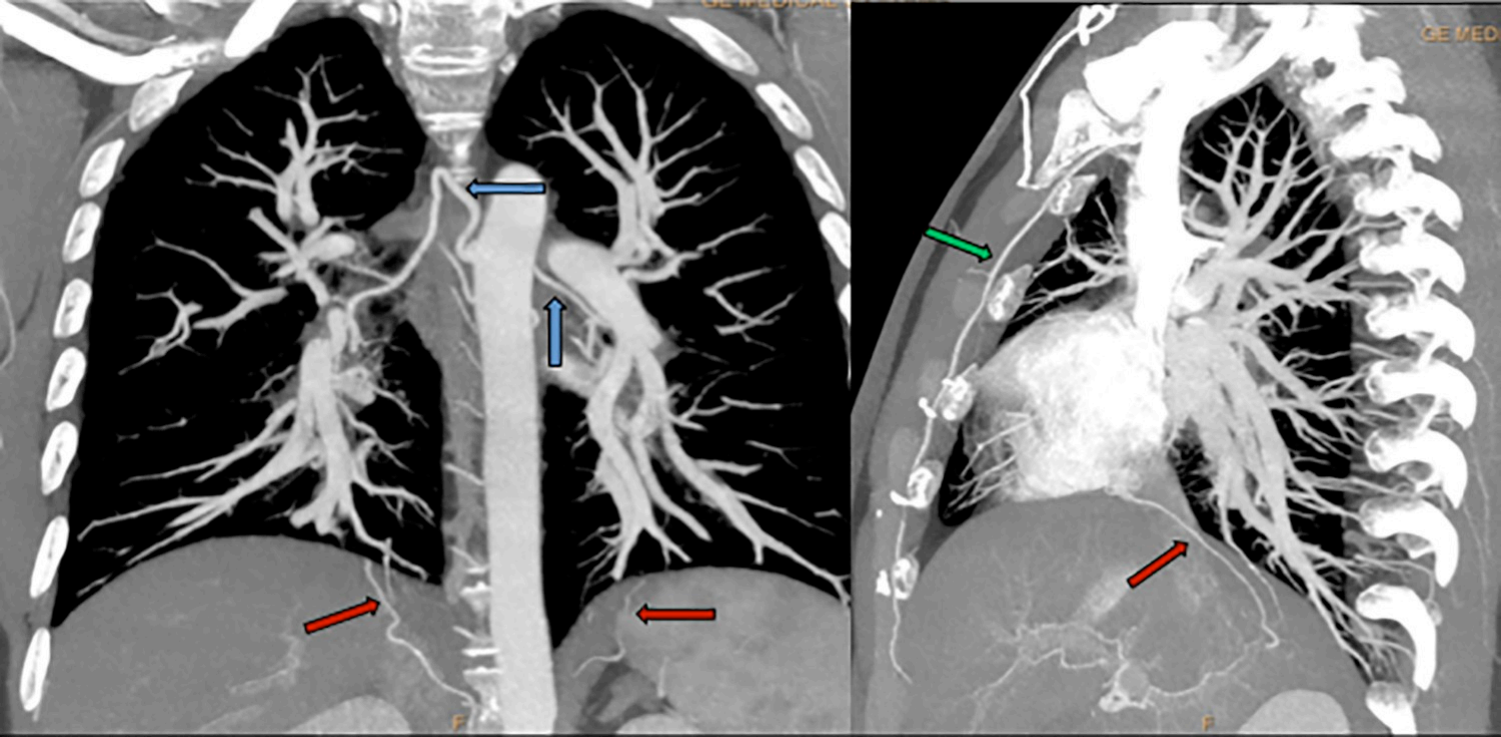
Multidetector Computed Tomography patient 5. Hypertrophy in mammary (green arrows), phrenic (red arrows) and bronchial arteries (blue arrows).
